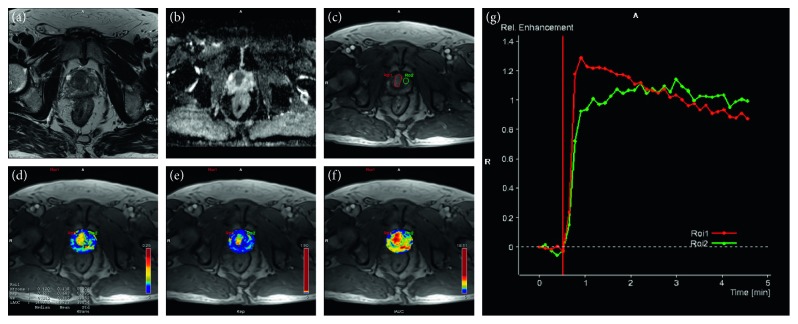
Transverse prostate MR images from a 69-year-old male patient with biopsy proven prostate cancer (Gleason score 3 + 4 and serum PSA 6.6 ng/mL): (a) T2-weighted image showing in the transitional zone a hypointense area without clear border; (b) ADC map: transitional zone hypointense region with a clear border, with ADC value of 0.75 × 10^−3^ mm^2^/s; (c) T1-weighted image early enhancement map: the enhanced region of interest 1 (ROI1, red line) corresponds to the tumor, and ROI 2 (green line) was selected from normal prostate tissue as healthy control; (d) *K*^trans^ map: ROI 1 *K*^trans^ 0.120 min^−1^ and ROI 2 *K*^trans^ 0.048 min^−1^; (e) *K*_ep_ map: ROI 1 *K*_ep_ 0.657 min^−1^ and ROI 2 *K*_ep_ 0.327 min^−1^; (f) iAUC map: ROI 1 iAUC 14.976 mmoL/L/min and ROI 2 iAUC 6.871 mmoL/L/min; (g) enhancement kinetics pattern from the two ROIs: the time-intensity curves were obtained from dynamic contrast-enhanced MRI. ROI1 showing a higher peak enhancement and an early wash-in and wash-out of contrast medium compared with ROI 2.
